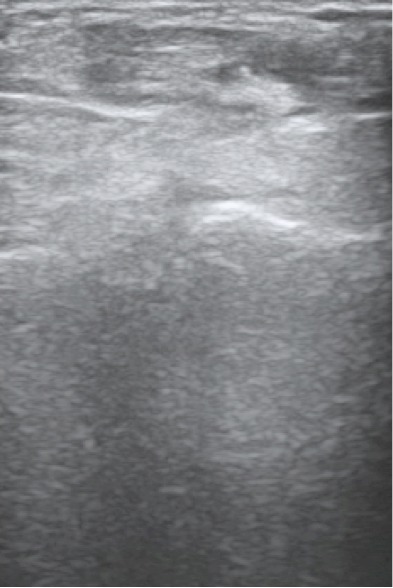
Ultrasonography wrist showing: tenosynovitis at the intersection of radial extensor tendons (ECRB, ECRL) with the abductor pollicis longus (APL) and extensor pollicis brevis (EPB)
